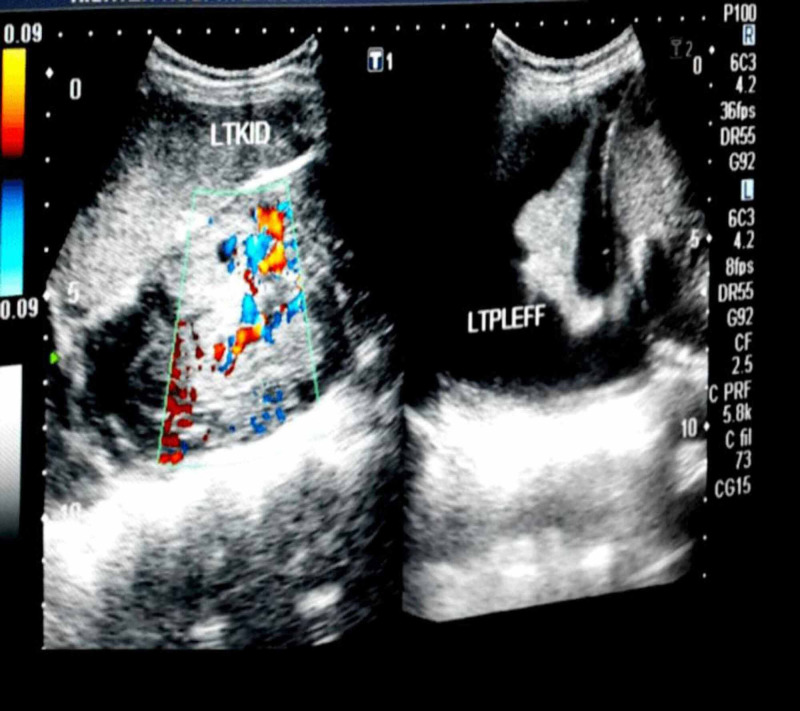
Ultrasound abdomen showing a vascular mass in the left kidney and a left pleural effusion
